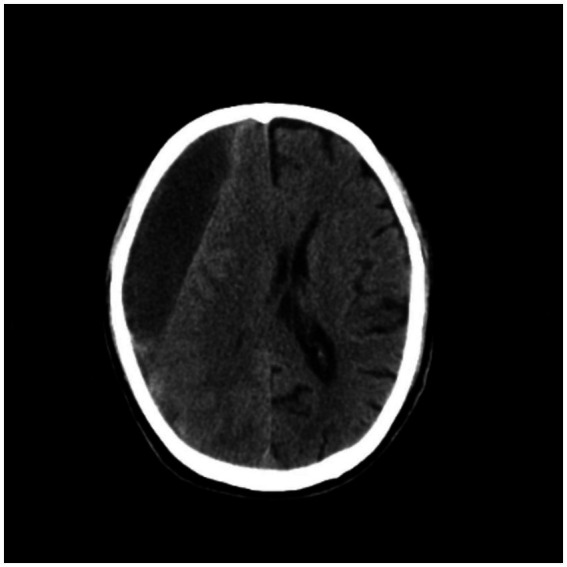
Preoperative brain CT revealed a crescent-shaped low-density shadow in the right frontotemporal parietal area, compression of the lateral ventricle, and a 0.8 cm shift to the left of the midline structure.
